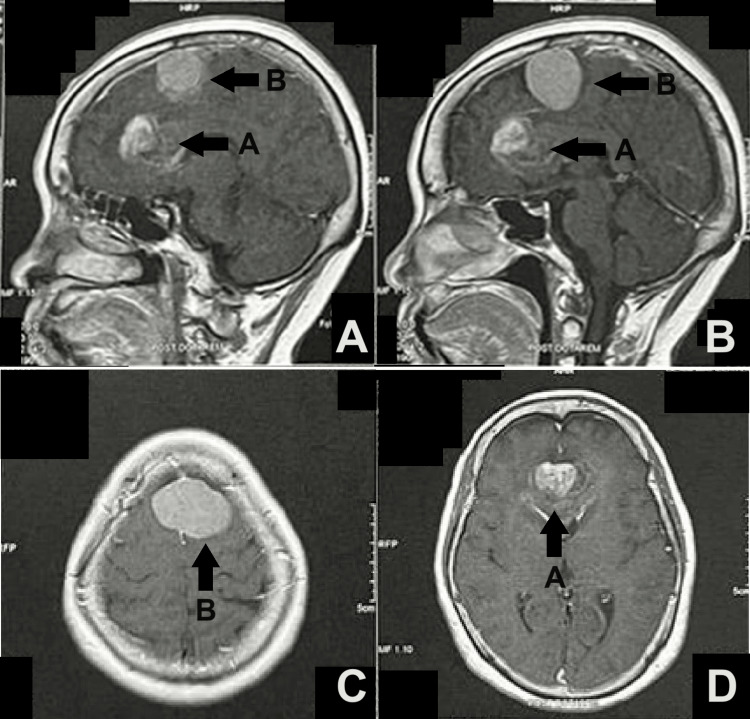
Cranial MRI of the patient, with and without contrast. The sagittal view in panels A and B shows arrow A pointing to an irregularly shaped butterfly tumor involving the callosal genu and the cingulate gyri, measuring 3.2x4.3x3.2 cm with a moderate amount of infiltrative edema. The axial view in panels C and D shows arrow B pointing to a separate homogeneously enhancing extra-axial mass, noted to straddle the high frontal interhemispheric falx, measuring 3.4x4.6x3.5 cm, appearing to infiltrate the middle third of the superior sagittal sinus and demonstrated no significant vasogenic edema.
